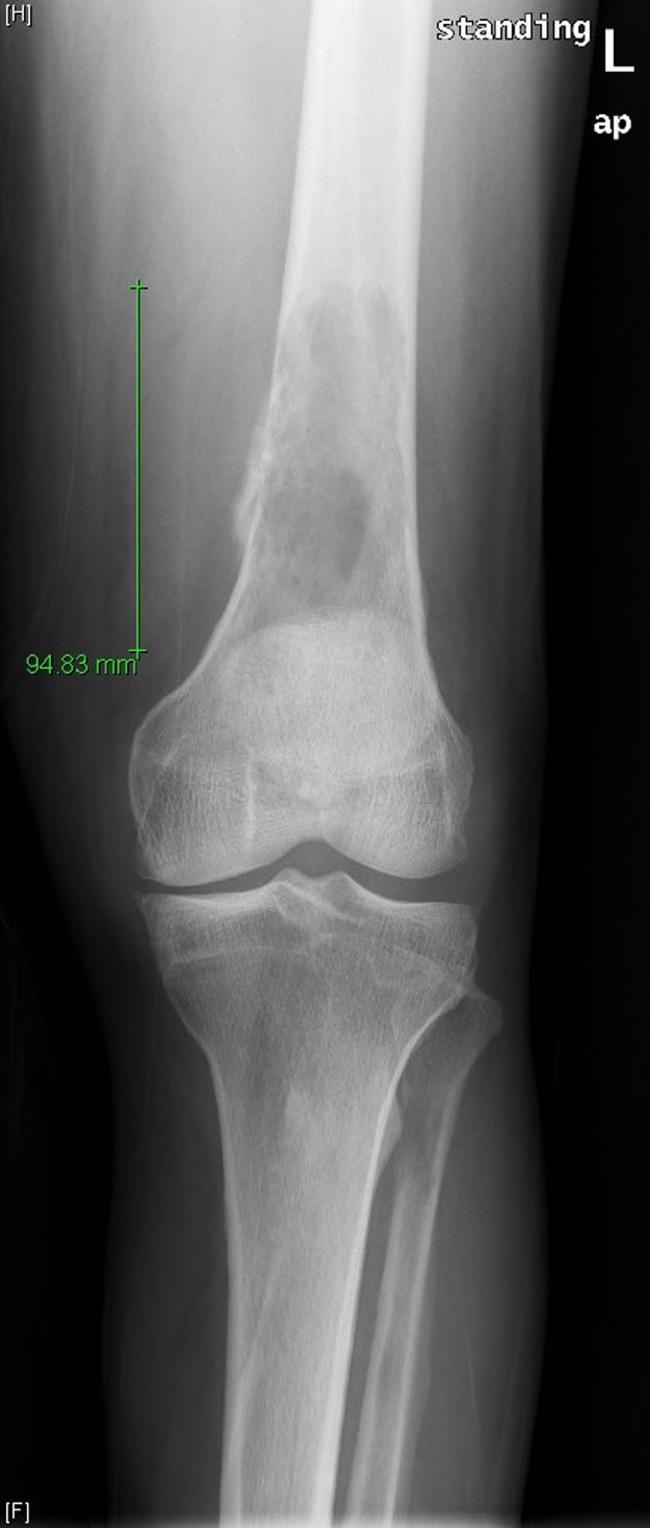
Frontal radiograph of the left femur demonstrates a large lytic lesion of the distal diaphysis with wide zone of transition and aggressive-appearing periosteal reaction at the medial margin.
